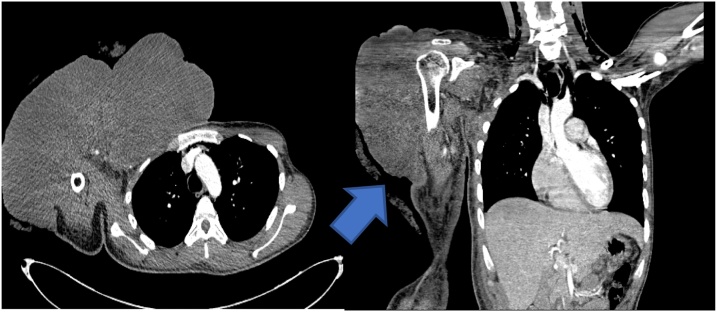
Thorax CT scan showing the tumor mass.
